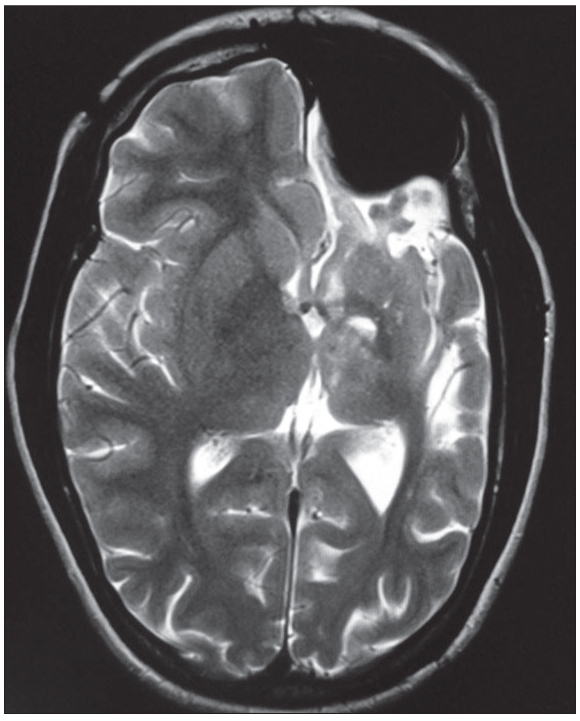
Axial T2-weighted image: Left cerebral hemiatrophy, ipsilateral occipital horn dilatation, ipsilateral midline shift, hypoplasia of thalamus, caudate nucleus, and lentiform nucleus are demonstrated. In addition, ipsilateral pneumosinus dilatans (frontal) is seen
